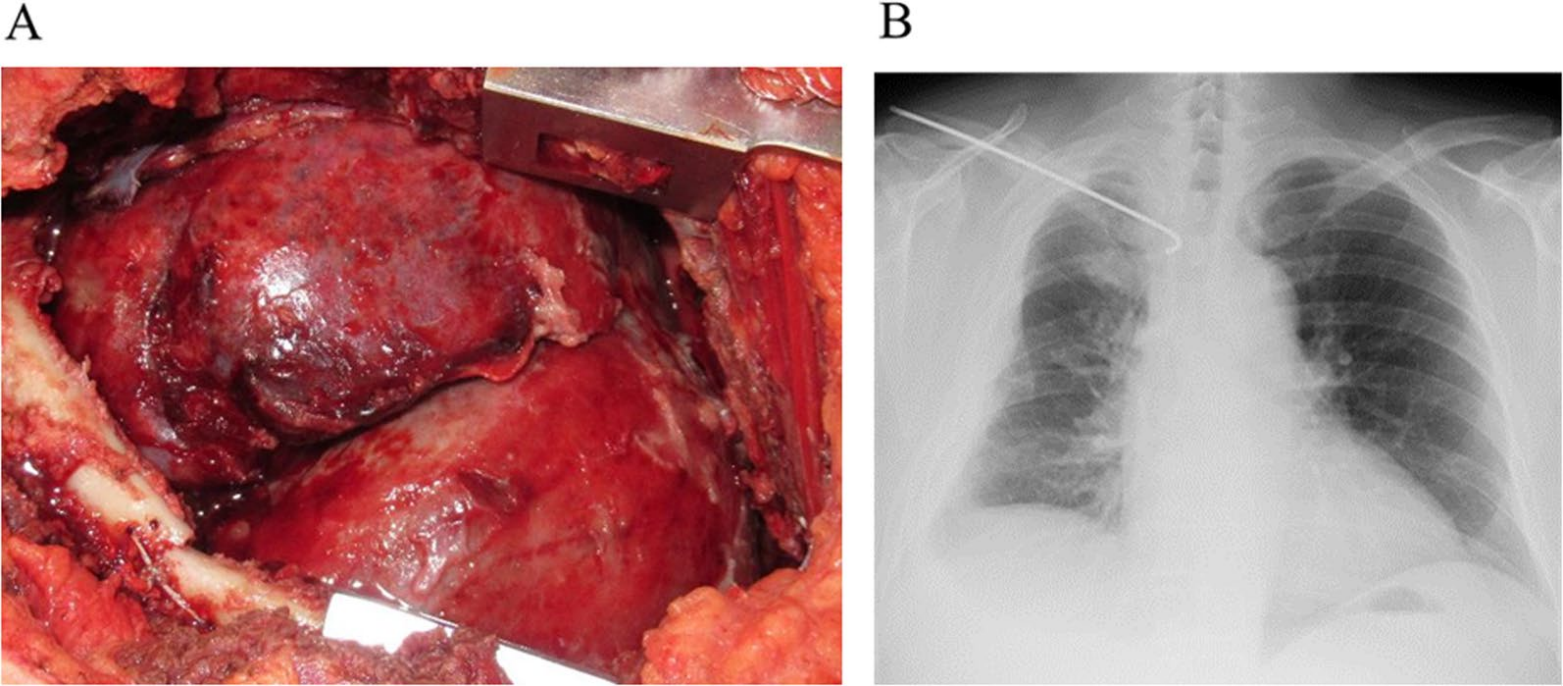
A The cavity of empyema with partial decortication after scraping and drainage. B Chest X-ray after surgery on postoperative day 16
